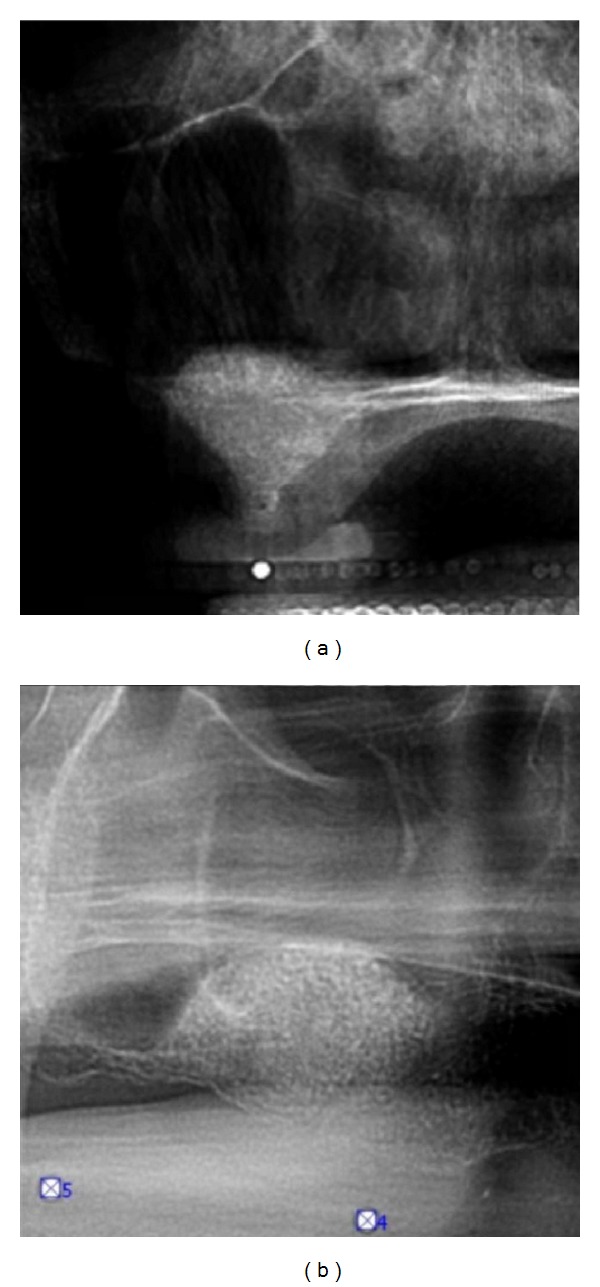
Transversal and parasagittal CBCT-scan 7 months post tHUCSL-Intralift. The ossification process is obviously completed.
